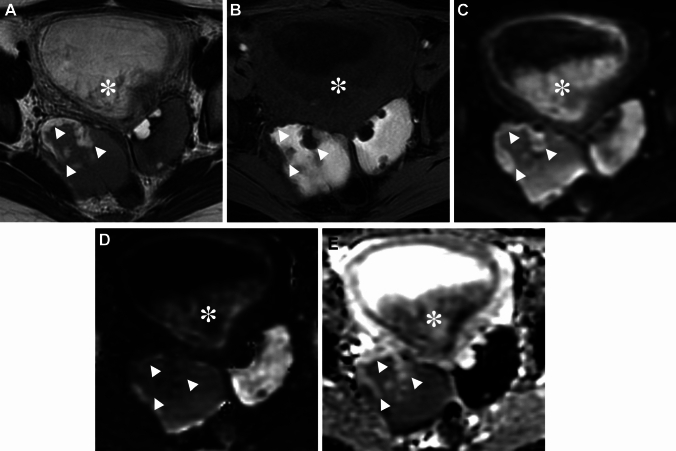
Decidualized endometrioma. A 32-year-old pregnant (13 weeks) with suspicion of an ovarian cystic mass with mural nodules on ultrasonography examination. A T2-weighted image, B fat-saturated T1-weight image, C DWI (*b* = 800 s/mm^2^). D computed DWI (*b* = 2000s/mm^2^), and E ADC map show a right endometrioma with multiple, flat mural nodules (arrowheads) exhibiting T2-prominent high signal intensity, T1-low signal intensity, and high signal intensity on DWI with high ADC (T2 shine-through) similar to those of the placenta (*). On the high b-value (*b* = 2000s/mm^2^) computed DWI shows the signal decrease of the mural nodules
